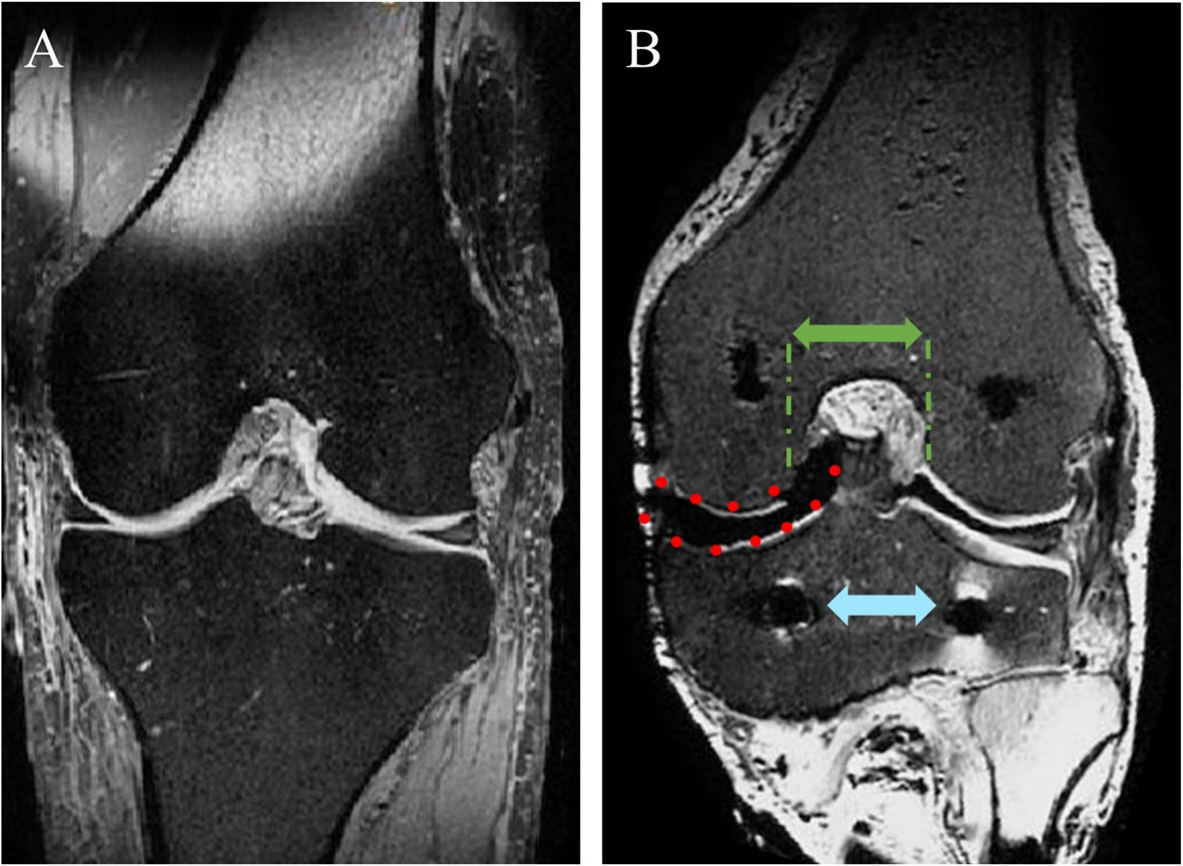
MRI scans in the frontal view (A–P): A) intact left knee joint and B) resected, with capsuloligamentous structures intact in the left knee joint (white tissue capsule), applied notchplasty (green arrow) with muscle insertion sites (blue arrow, hamstring muscles) and device left in place (red dashed line, black shadow)
